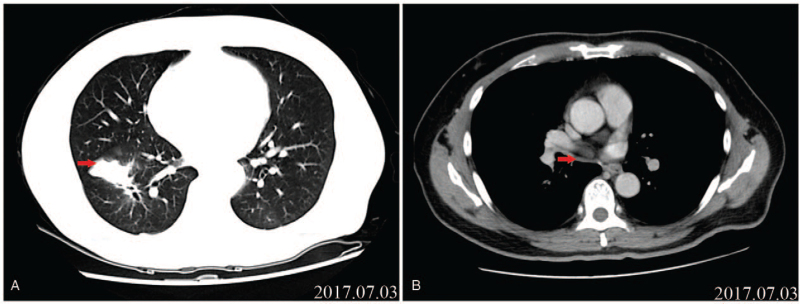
Computed tomography (CT) scan. (A) Chest scan indicating the presence of a nodule of 3.1 × 2.4 cm in the right lower lobe indicating the decrease in its mass after the use of gefitinib as neoadjuvant treatment. (B) Subcarinal lymph node metastasis disappearance after the use of gefitinib as neoadjuvant treatment.
